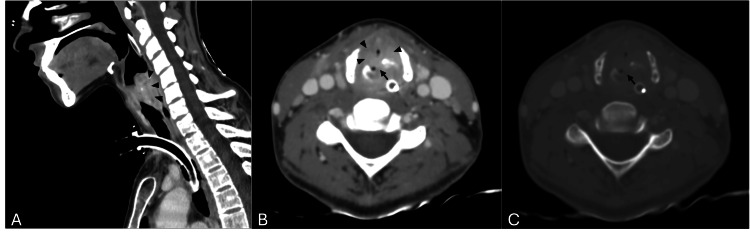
Neck CT after tracheostomy (A) Sagittal and (B, C) axial computed tomography images demonstrating a displaced, vertically oriented complete fracture of the cricoid cartilage (arrow), associated with a subglottic abscess (arrowheads).
